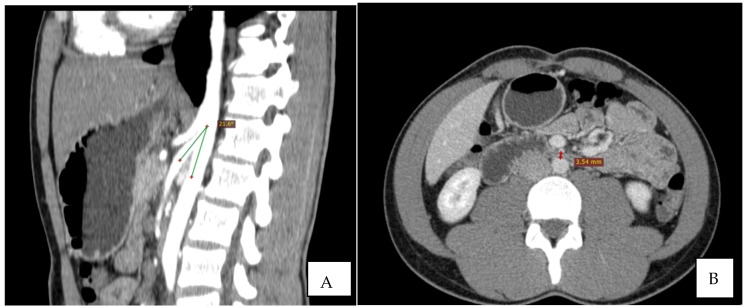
CT: the sagittal reconstruction shows the reduction of aorto-mesenteric angle. ((A) beak signand distance (B)).
